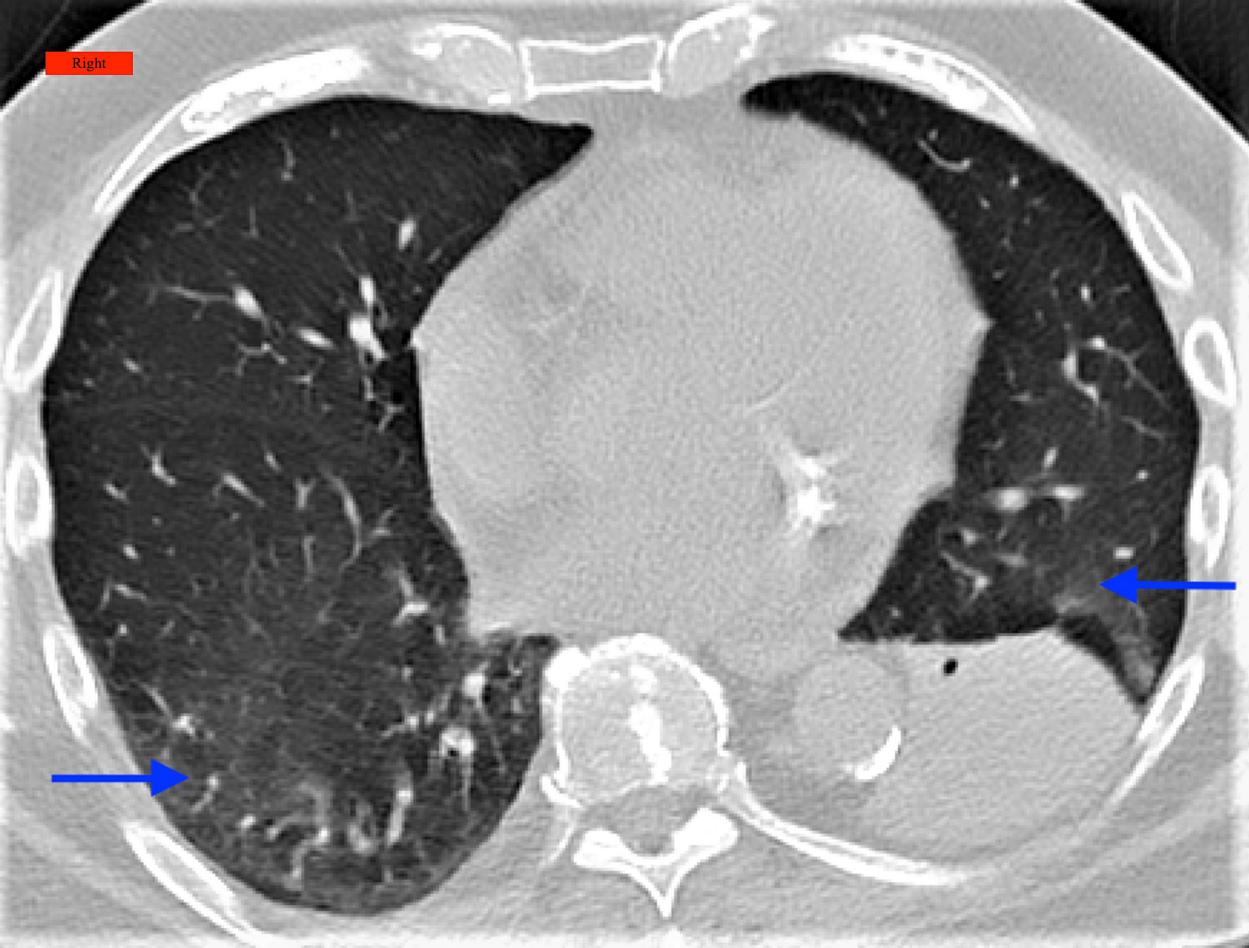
CT chest showing consolidation and volume loss in the left lower lobe and mild ground-glass opacities bilaterally (arrows) Right: Indicates the right side of the patient; CT: Computed Tomography
